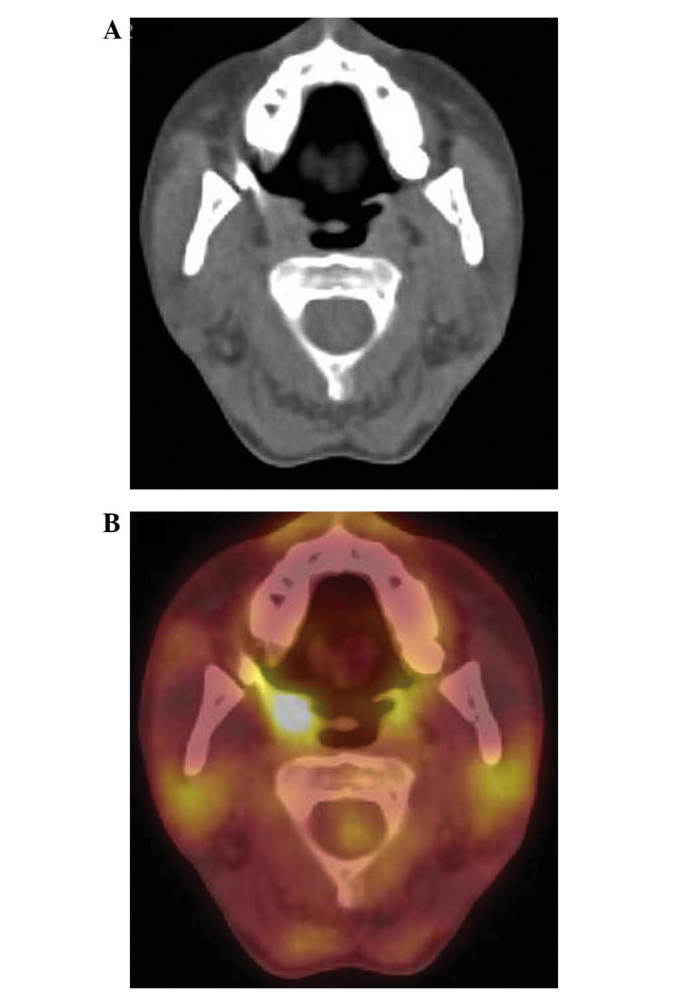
(A) No tumor was visible on the CT axial scan. (B) PET CT axial scan revealed a tonsillar tumor (right side). CT, computed tomography; PET, positron emission tomography.
